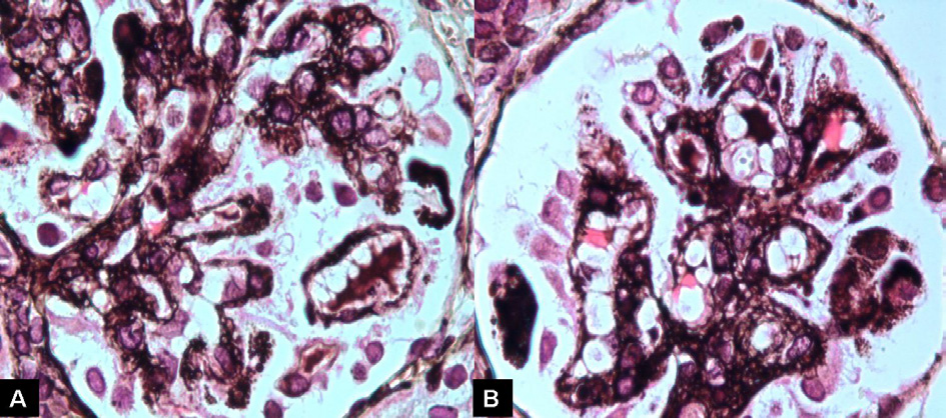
Her skeletal survey was normal. The magnetic resonance imaging (MRI) of brain was done and is shown in Figure 4. Genetic testing revealed no mutations in *NPHS1* and *NPHS2* genes. Her cytogenetic analysis could not be done due to non-availability of the test.
